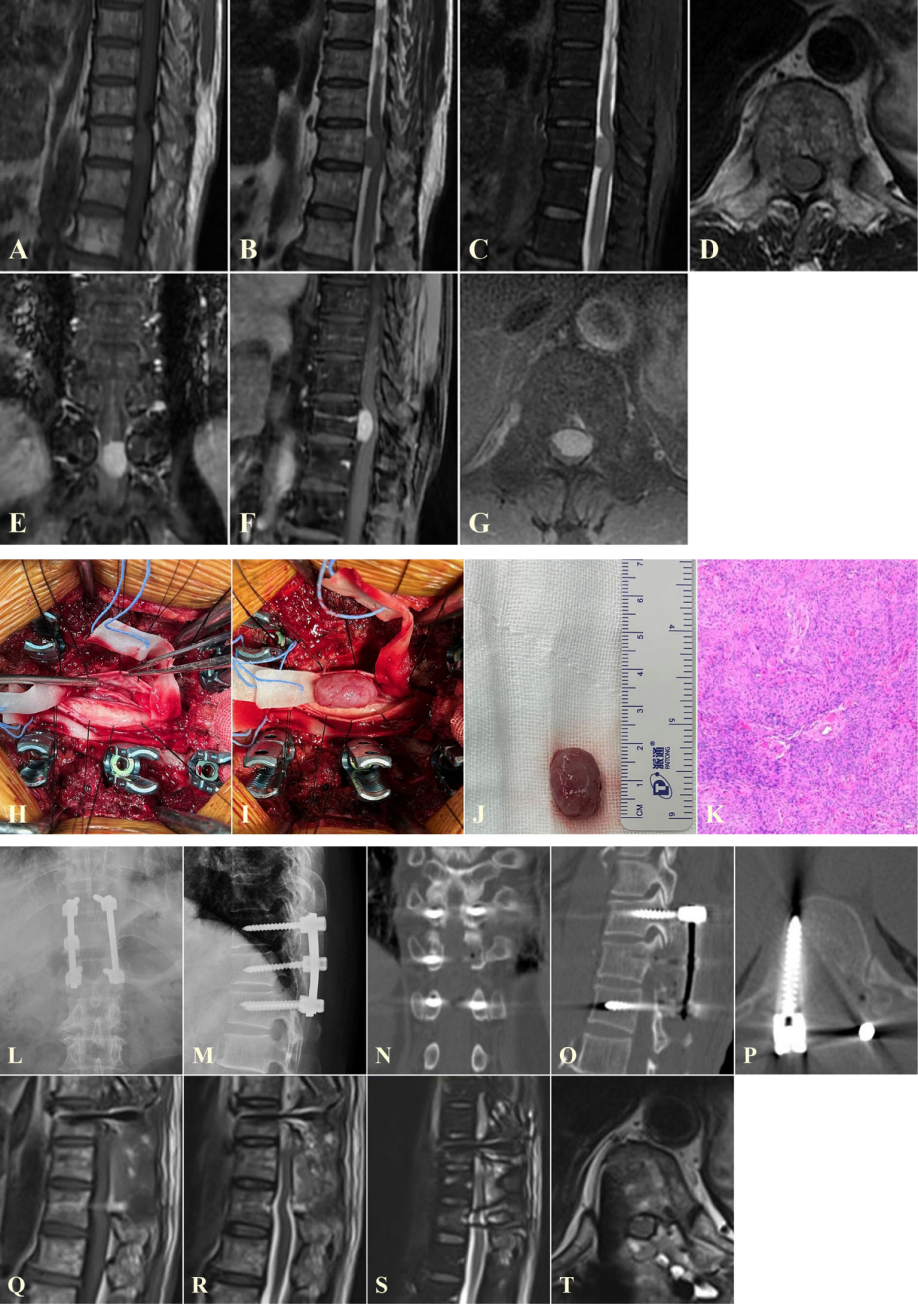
A representative case of one-piece resection for managing a VIESM at T11. (A–G) Preoperative MRI images showing the VIESM at T11. (H–K) One-piece resection of the VIESM during the operation, verified later by the pathological biopsy findings. (L–T) Postoperative X-ray (L, M), CT (N–P), and MRI (Q–T) images demonstrate the complete removal of the VIESM via one-piece resection and fine internal fixation. VIESM, ventral intradural extramedullary spinal meningioma; MRI, magnetic resonance imaging; CT, computed tomography.
